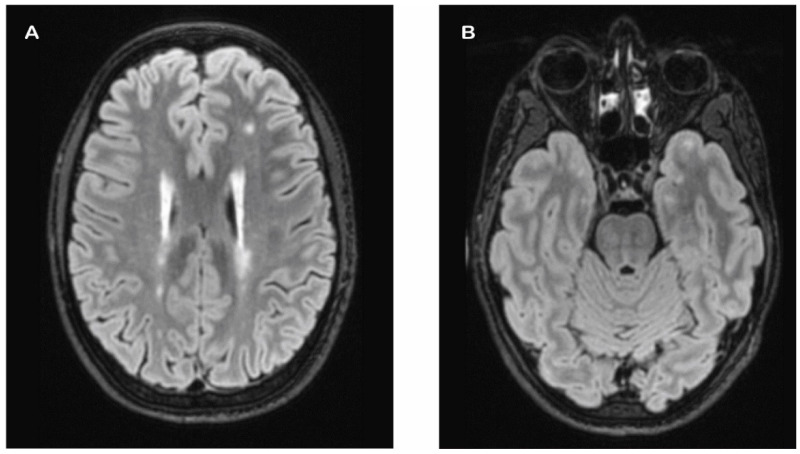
Patient 2’s FLAIR MRI axial plane showing multiple punctate hyperintensities in the deep white matter and confluent lesions in the periventricular white matter (A) and minimal involvement of anterior temporal poles (B).
